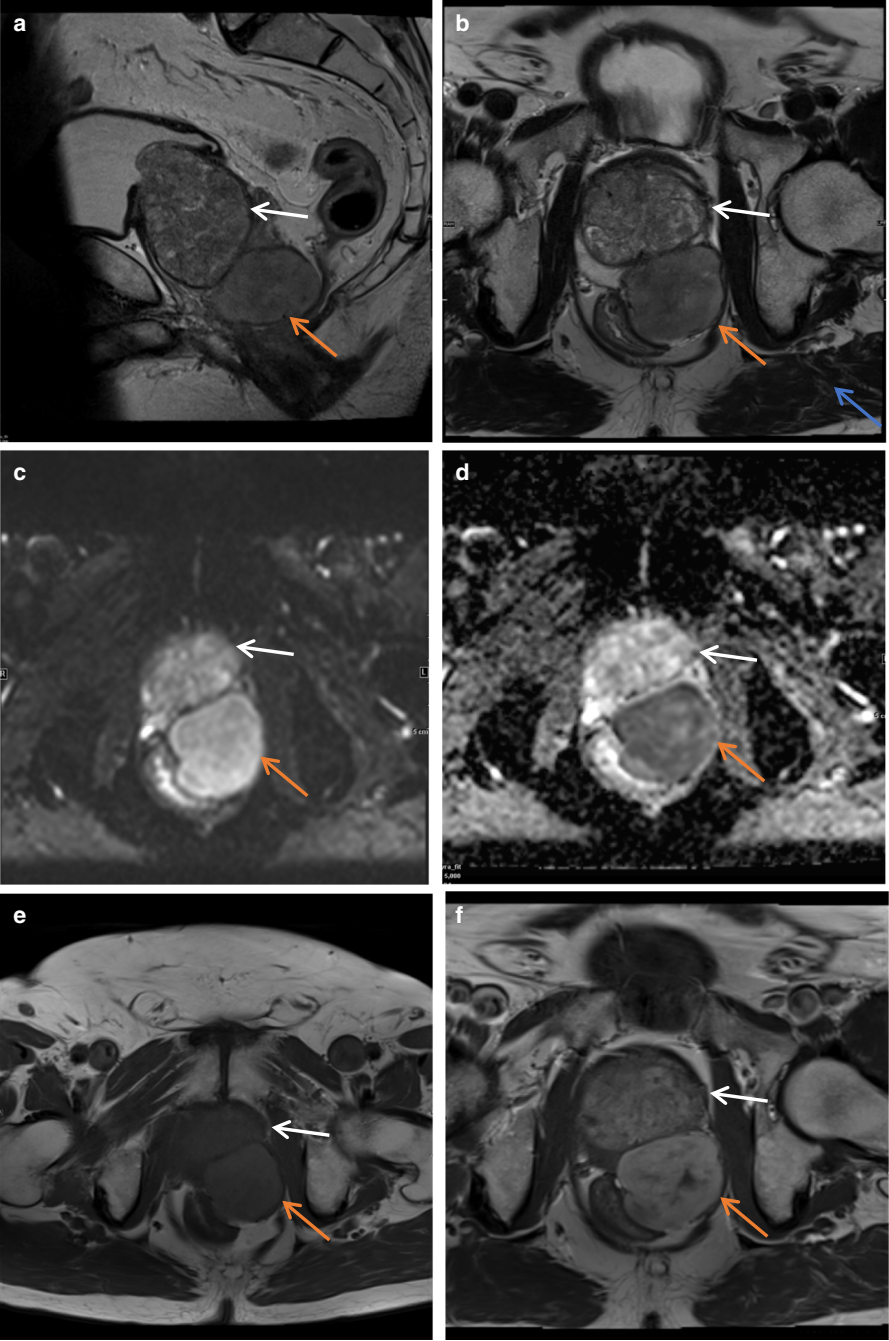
Solitary fibrous tumour* A well-circumscribed homogeneous lesion (red arrow) arising from the left posterolateral prostate wall is seen in (a) sagittal and (b) axial T2W images as isointense to prostate (white arrow) and hyperintense to gluteus muscle (blue arrow). c DWI and (d) ADC images demonstrate diffusion restriction in the lesion. In e and f the lesion is iso- to mildly T1 hyperintense to prostate and shows uniform contrast enhancement. This was biopsy-proven solitary fibrous tumour
